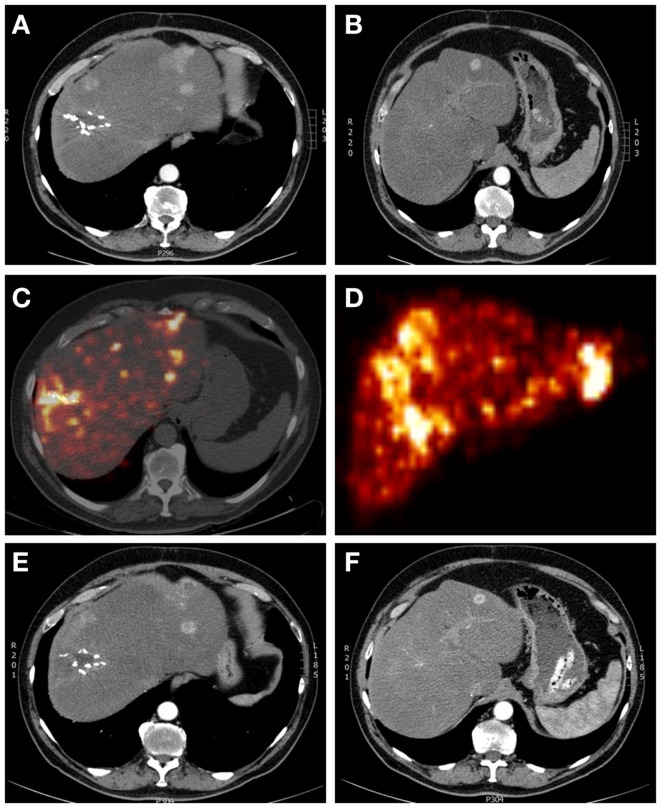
A 65-year-old male patient with multifocal recurrent hepatocellular carcinoma (HCC) 6 months after resection of a HCC in liver segment 7–8. (A,B) Arterial phase contrast-enhanced CT showing operation clips *in situ* after resection of the primary HCC and multiple sites of contrast enhancement (with wash-out on delayed images – not shown). (C) Yttrium-90 PET-lowdose CT and (D) maximum intensity projection after treatment with 1.7 GBq ^90^ Y labeled SIR-Spheres, showing markedly increased uptake of SIR-Spheres at the site of resection and multifocal in both right and left liver lobes, with clear right-sided predominancy. (E,F) Arterial phase contrast-enhanced CT showing response with central necrosis in tumor lesions, with no significant changes in liver volume.
